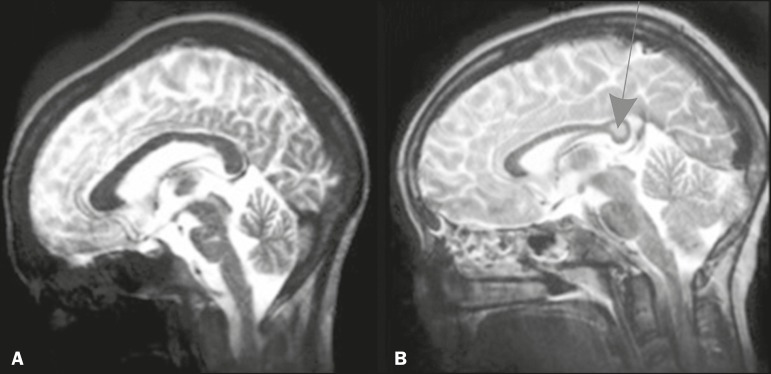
MRI scans of the skull in several patients diagnosed with systemic lupus
